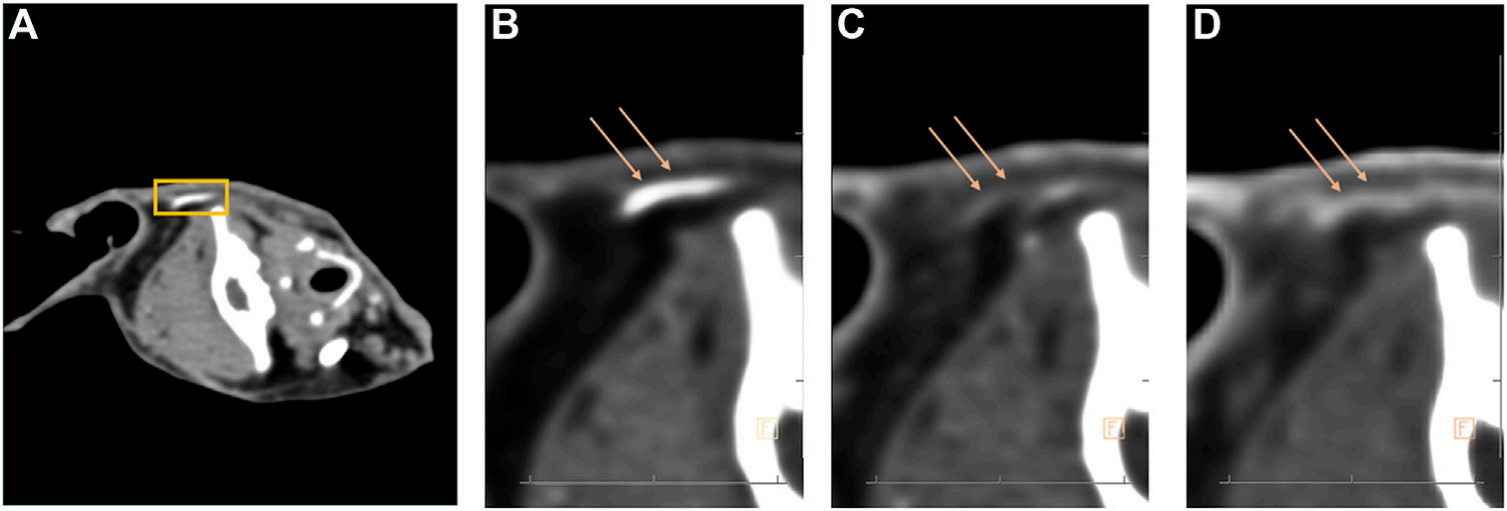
Computed tomography scans the sagittal plane of the entire skull and the marginal ear artery was marked with a yellow square (Figure 2A). The auricular artery is filled with blood vessels (Figure 2B), and the blood vessels are significantly constricted after administration of ephedrine (Figure 2C). The diameter of the marginal auricular artery partially recovered within 30 min (Figure 2D). The loss of visibility of blood vessels due to narrowing of blood vessels and insufficient filling of contrast agent (Figure 2C).
